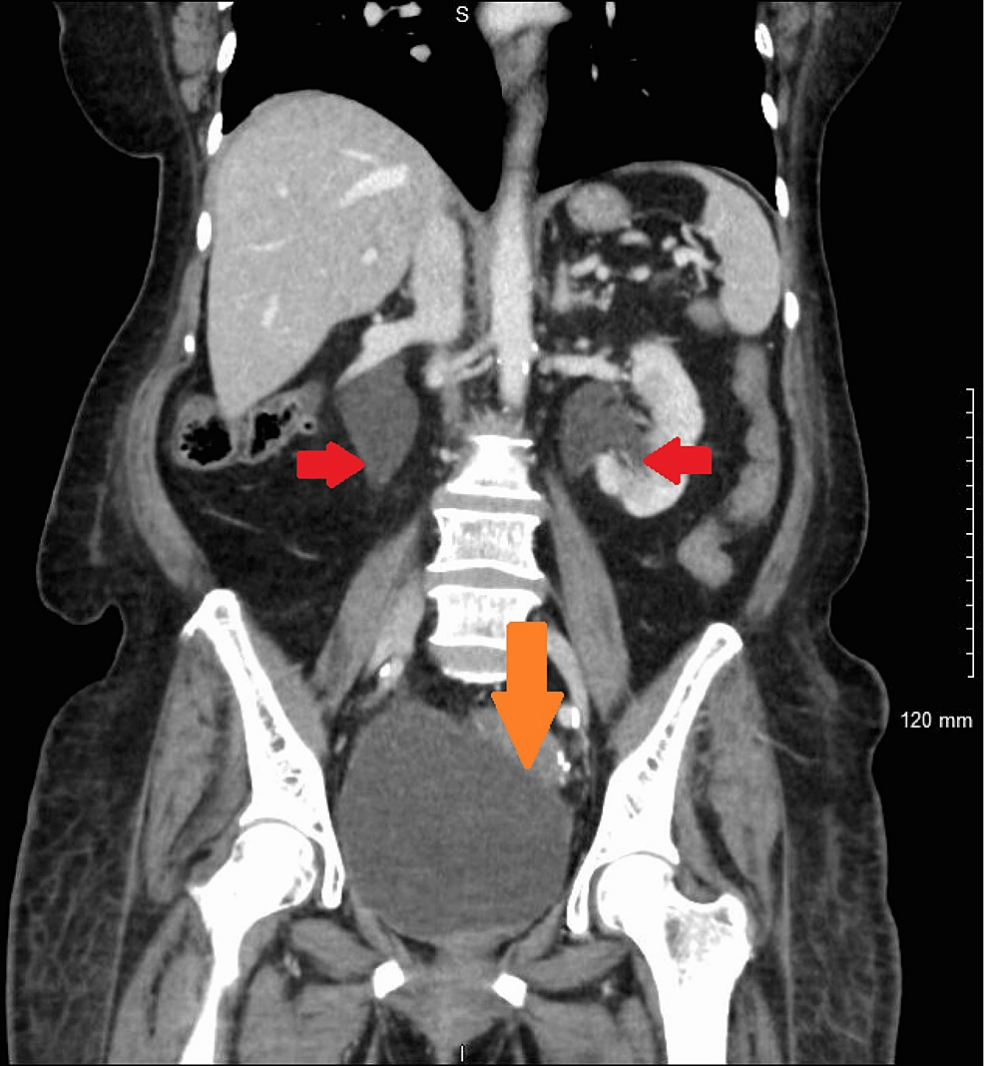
Bilateral hydroureteronephrosis (red arrows) and distended bladder (orange arrow) noted on computed tomography of the abdomen and pelvis.
